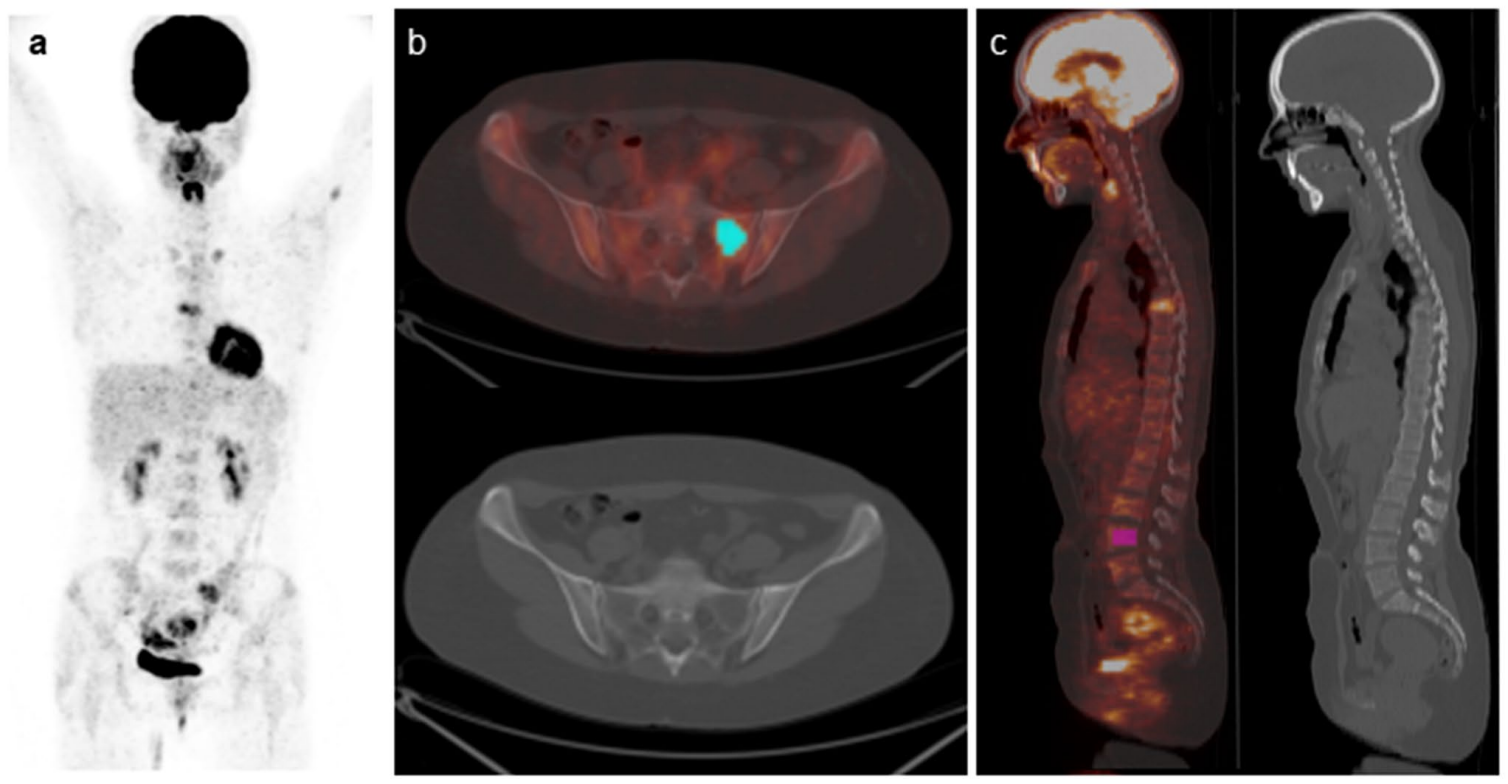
Representative images of 18F-FDG PET/CT scan in a MM patient. (a) Maximal intensity projection PET image; (b) transaxial fusion images of co-registered PET and CT showing the segmentation (light blue) of the hottest focal lesion; (c) sagittal fusion images of co-registered PET and CT showing the segmentation (pink) of a lumbar vertebral body for assessing diffuse bone marrow involvement
